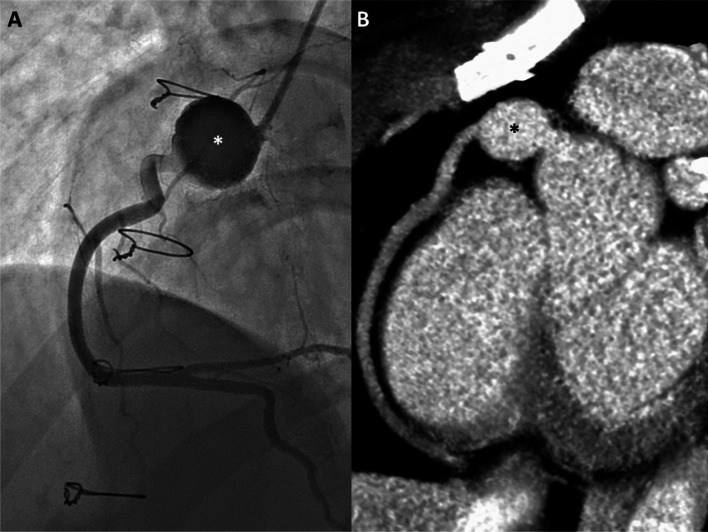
Pseudo-thrombus at catheterization. A) Right coronary injection demonstrates a large proximal aneurysm with an apparent central oval lucency (white asterisk). This was interpreted as a ball-valve thrombus in this young man with chest pain symptoms. B) Same-day coronary CT acquired 1 minute after injection shows completely uniform iodine intensity within the aneurysm (black asterisk), confirming the absence of any real thrombus – indicating that the catheter finding was the result of swirling flow and incomplete mixing of blood and iodine immediately after injection.
